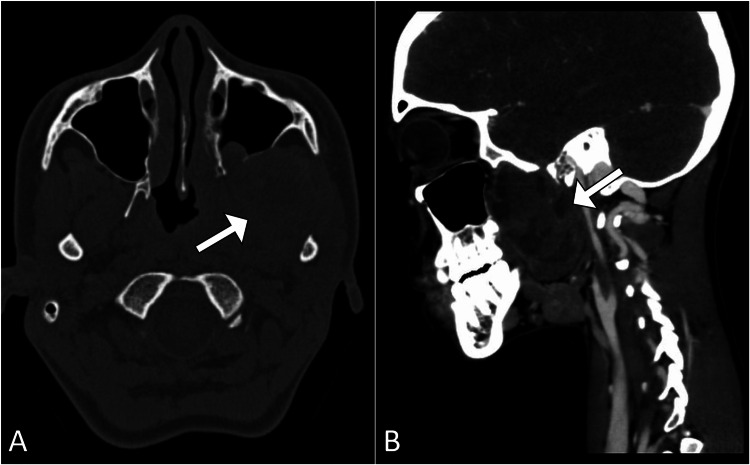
(A) CT scan of the brain demonstrates a soft tissue lesion (arrow) situated in the petrous part of the left temporal bone. (B) After administering contrast, the lesion demonstrates heterogeneous enhancement. CT: computed tomography
